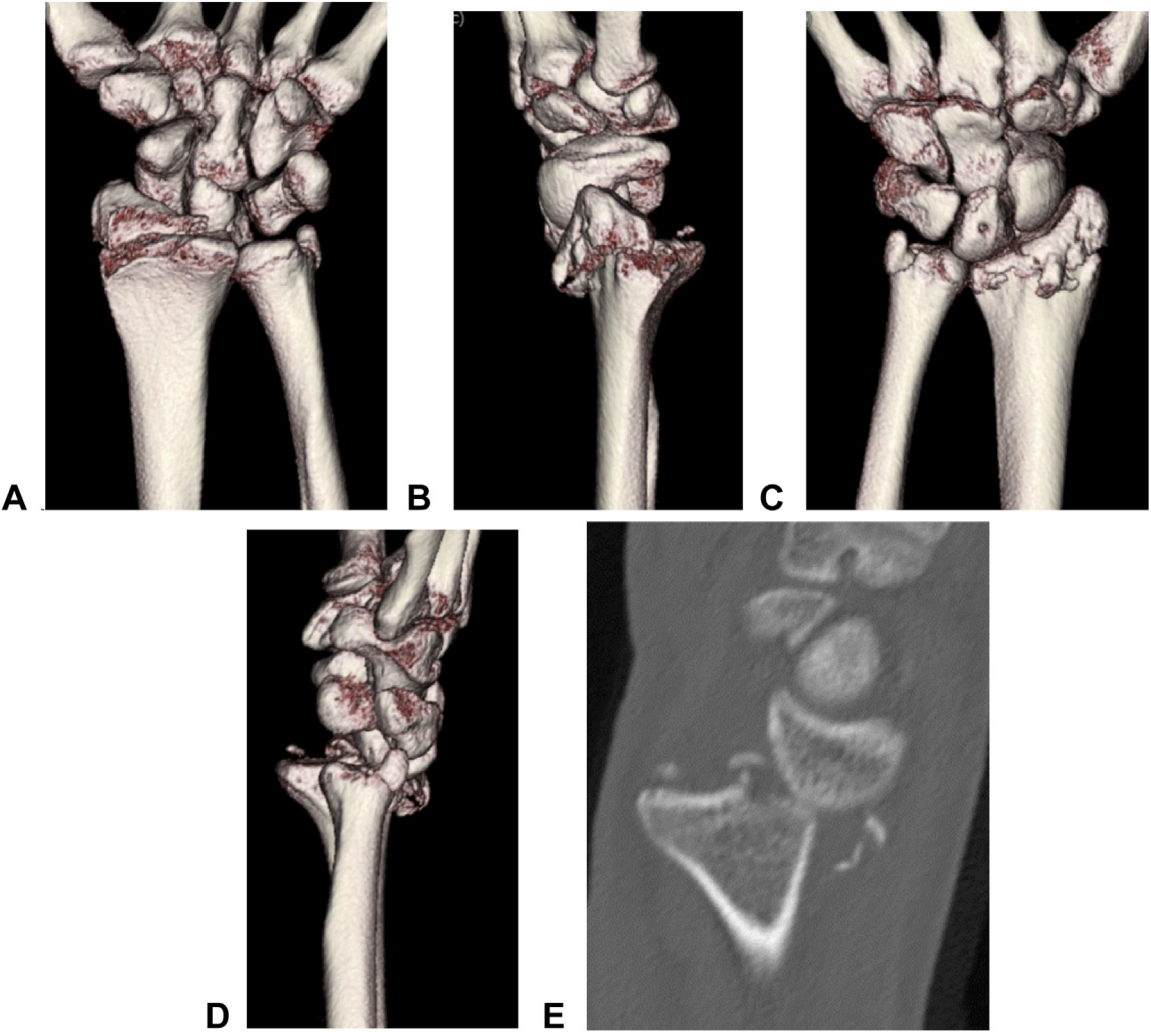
Three-dimensional computed tomographic reconstructions of the left wrist. A Palmar view. B Radial view. C Dorsal view. D Ulnar view. E Sagittal reformation of computed tomography confirms a distal radial fracture, including a radial styloid fracture, dorsal rim avulsion fracture, and dorsal dislocation of the radiocarpal joint.
